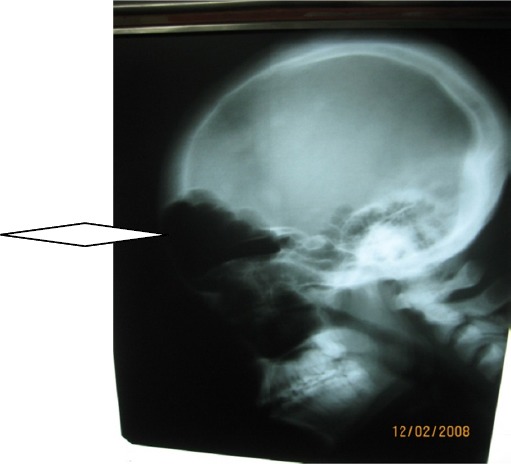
Lateral skull radiograph showing enlarged frontal and maxillary sinuses
